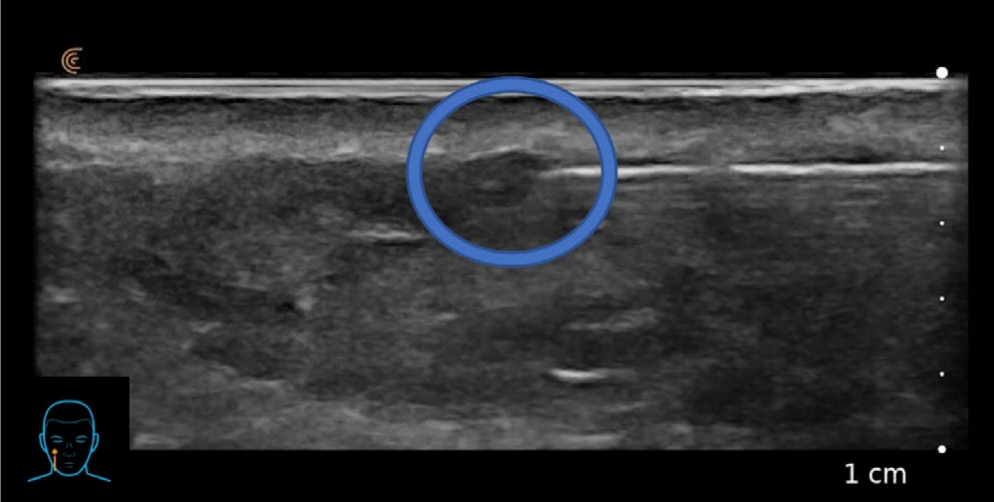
Subject's superficial HA placement on ultrasound during subdermal linear‐thread injection of CPM‐HA20G using a 25G 38 mm cannula.
